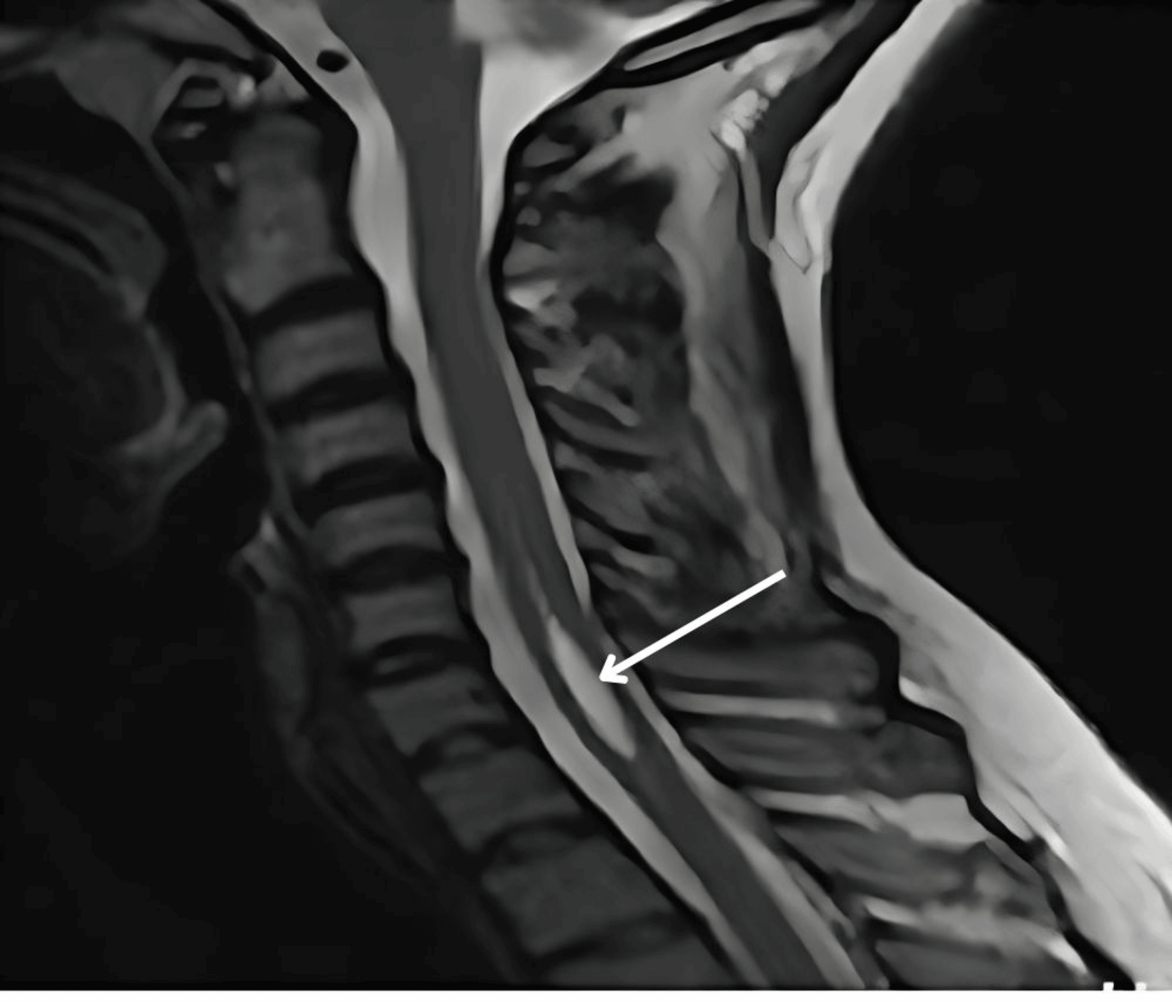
MRI sagittal T2-weighted sequence of the spine in Type 0 Chiari malformation showing a fluid-filled cavity in the cervical region (white arrow), without evidence of herniation of the cerebellar tonsil.
